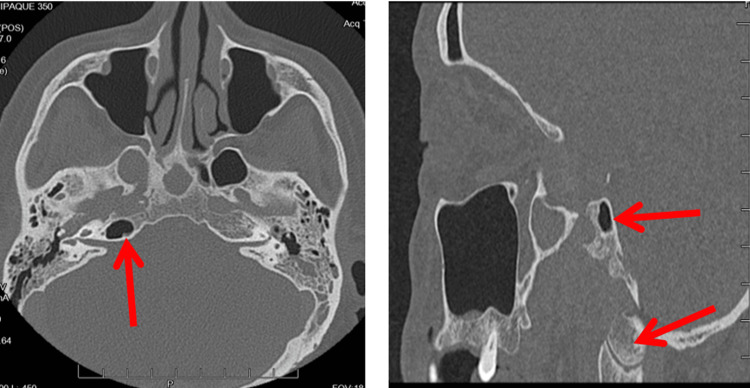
(Left and right) Repeat CT showing continued osseous erosion of the right petrous temporal bone now spreading to the clivus and right occipital condyle. Red Arrows: osseous erosion
